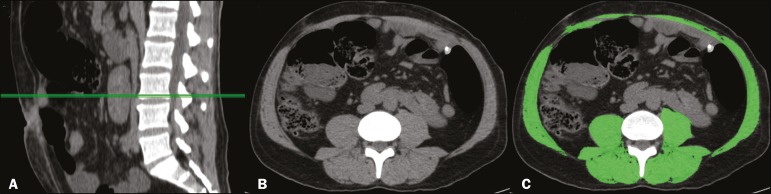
CT measurements of lean body mass. A: Sagittal plane used in order to set the axial plane at the level of the inferior endplate of the third lumbar vertebral body (B). C: Segmentation of the lean body mass at the same level as B.
